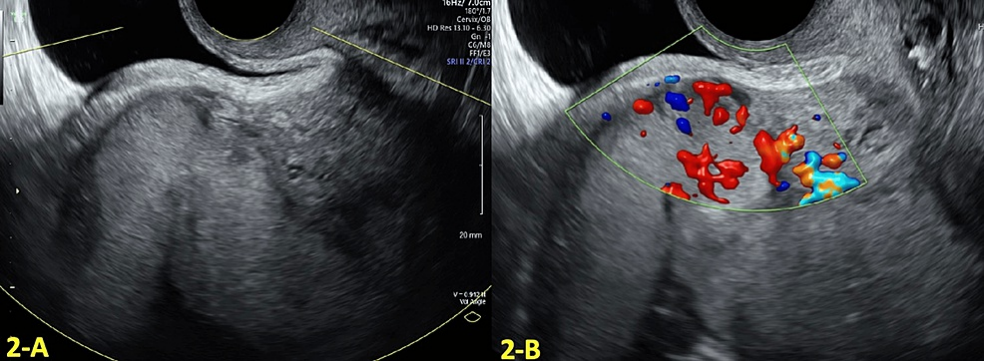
Transvaginal ultrasound (TVUS) findings at 18 weeks and six days gestational age (GA) A: Transvaginal ultrasound (TVUS) at 18 weeks and six days gestational age (GA) showing loss of the hypoechoic boundary of the myometrium in the lower uterine segment and placental bulge. B, Color Doppler at 18 weeks and six days GA showing increased vascularity of the uterine serosa-bladder interface.
